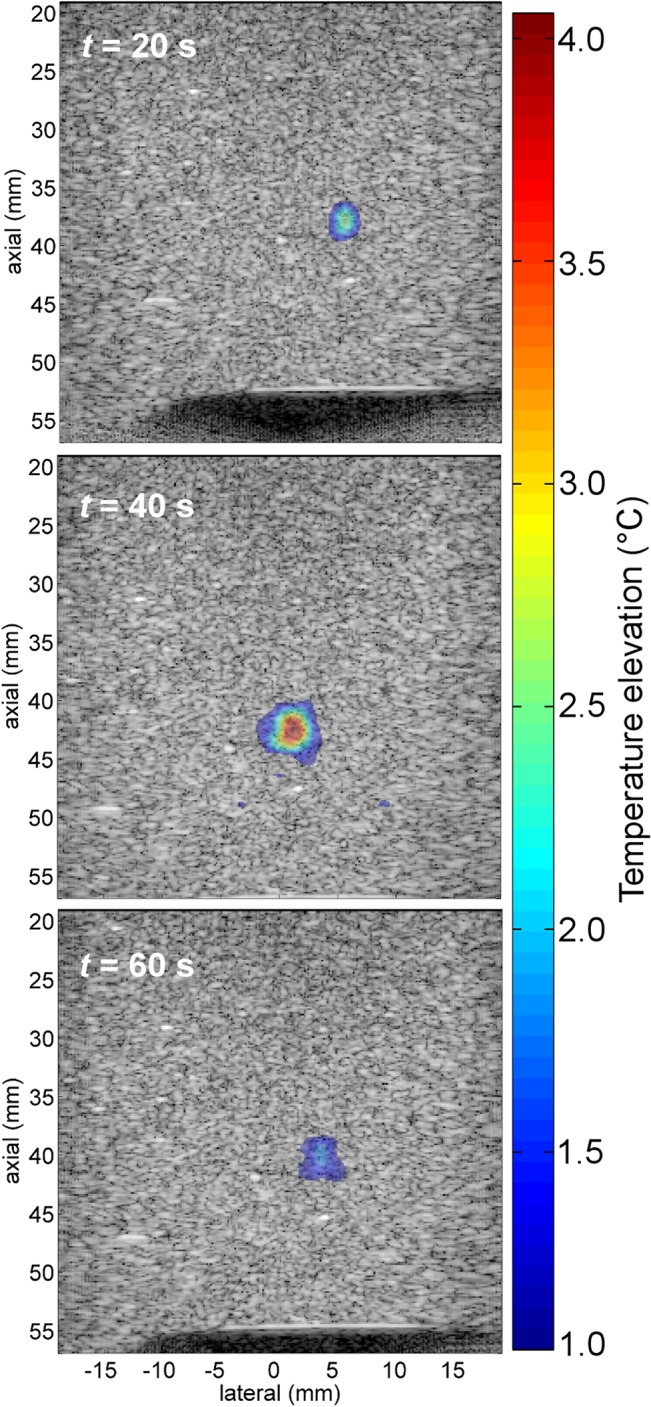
Temperature map and ultrasound imaging. Temperature map is superimposed on B-mode image at different times for 2-D diagonal motion with a total displacement of 7.1 mm. HIFU was activated for 30 s (between *t*
